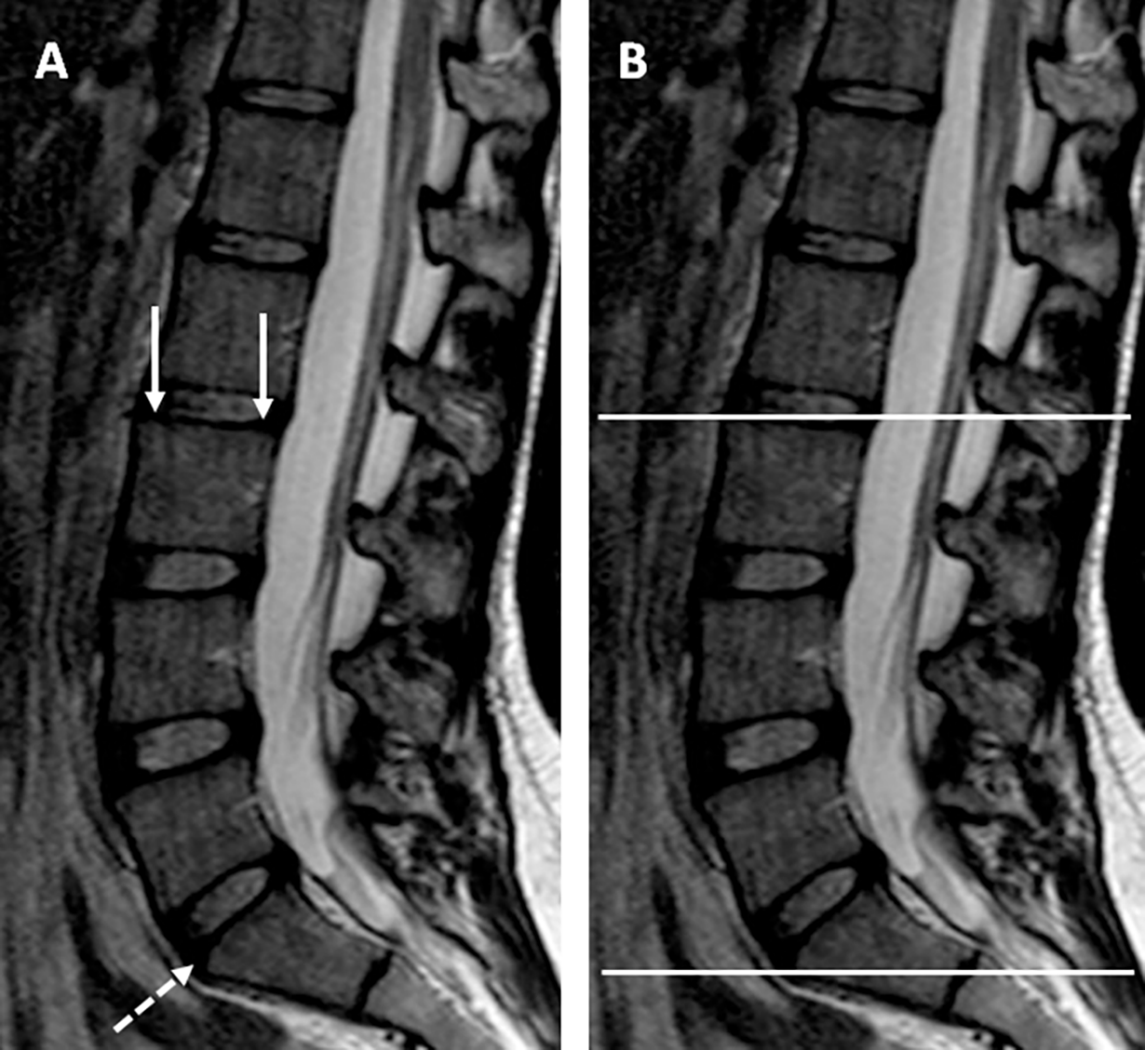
Sagittal T2-weighted image to demonstrate the superior and inferior limits of MRI axial slices acquisition. A) The white arrows point to the L3 superior endplate; the dashed arrow points the anterior margin of the S1 superior endplate. B) The white lines represent the upper and inferior limits of the block of axial slices.
